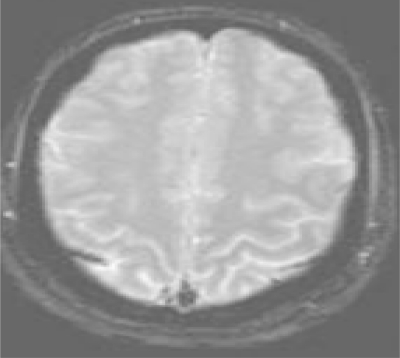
Cranial MRI- demonstrating occlusion of the sagittal sinus thrombosis.
